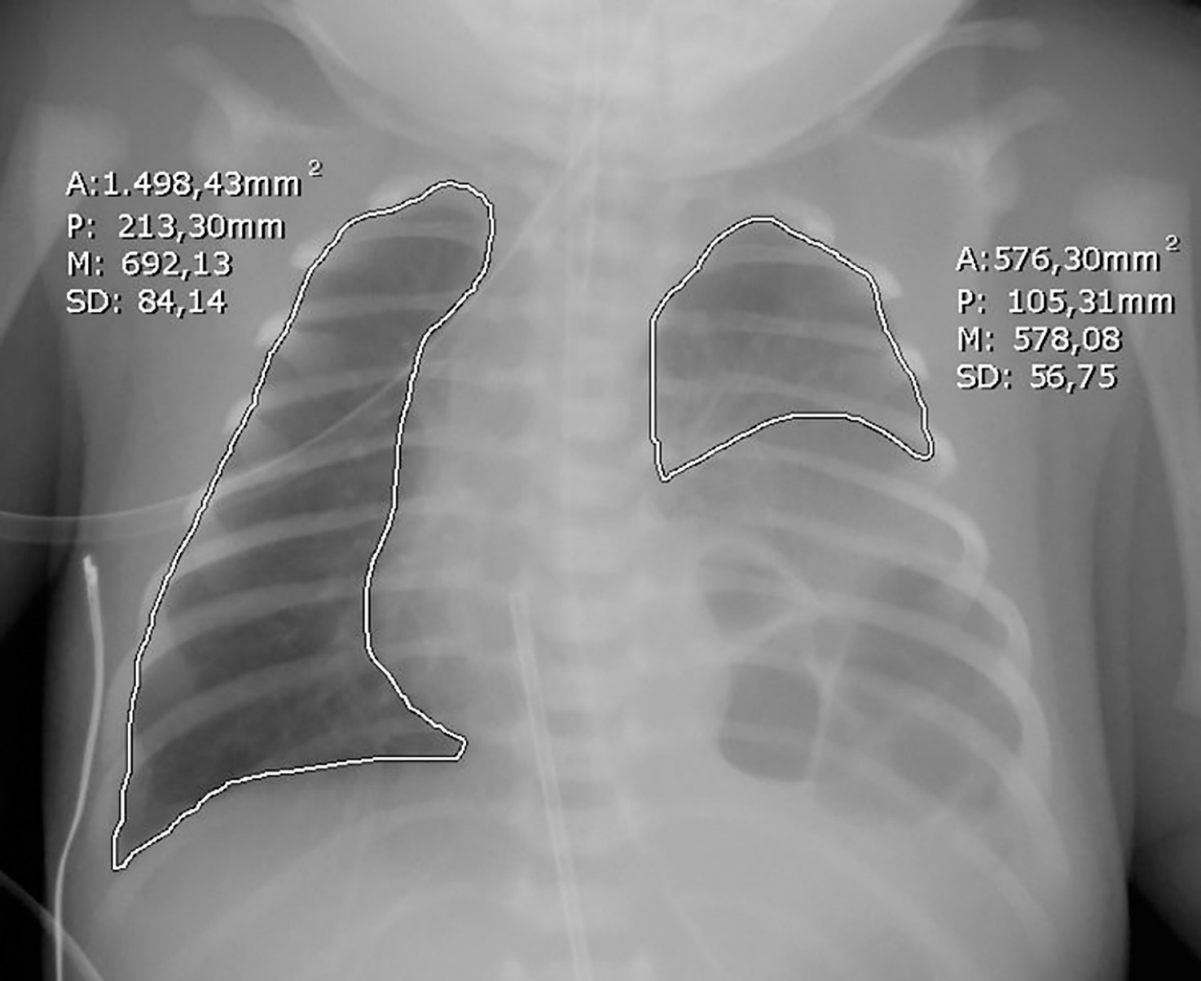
Chest radiographic pulmonary area. Calculation of the radiographic pulmonary area on neonatal chest x-ray.
